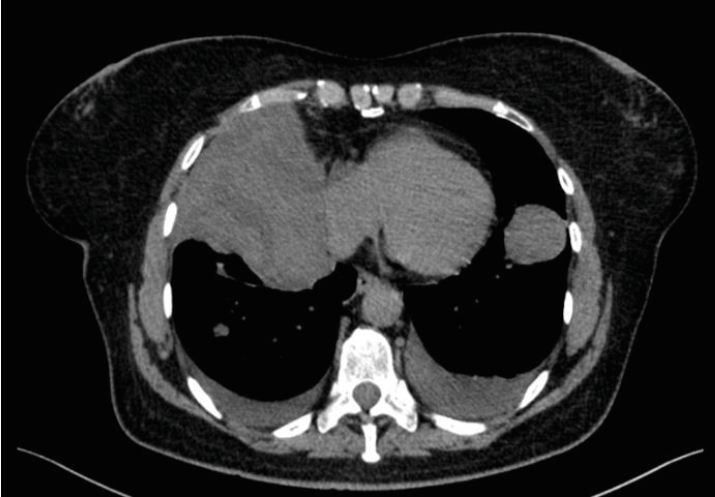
CT picture in the transverse plane. The finding at the time of diagnosis (12/2018).
